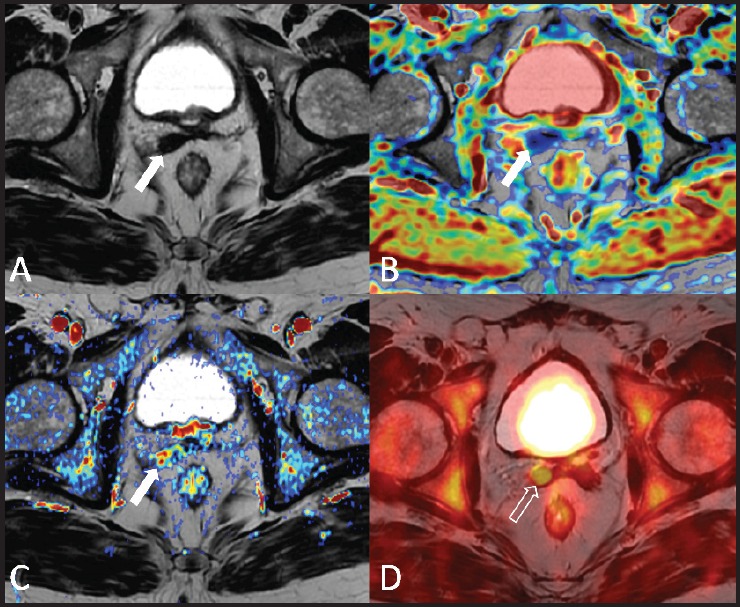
A 73-year-old patient who underwent radical prostatectomy 40 months earlier for prostate cancer Gleason 7 (3 + 4). Current PSA value of 2.2 ng/mL. The T2-weighted axial image (A) shows a hypointense nodular thickening of the remnant of the right seminal vesicle (arrow), which is characterised by restricted water diffusion on the apparent diffusion coefficient (ADC) map (B) and is hypervascular on the perfusion map obtained from the DCE–MRI acquisition (C) The ^18^F–choline–PET/MRI fused axial image (D) shows a focal accumulation of the tracer in correspondence to the remnant of the right seminal vesicle (void arrow), which confirmed the diagnosis of local relapse. Images obtained with Quanta Prostate, Camelot Biomedical Systems s.r.l., Genoa, Italy.
